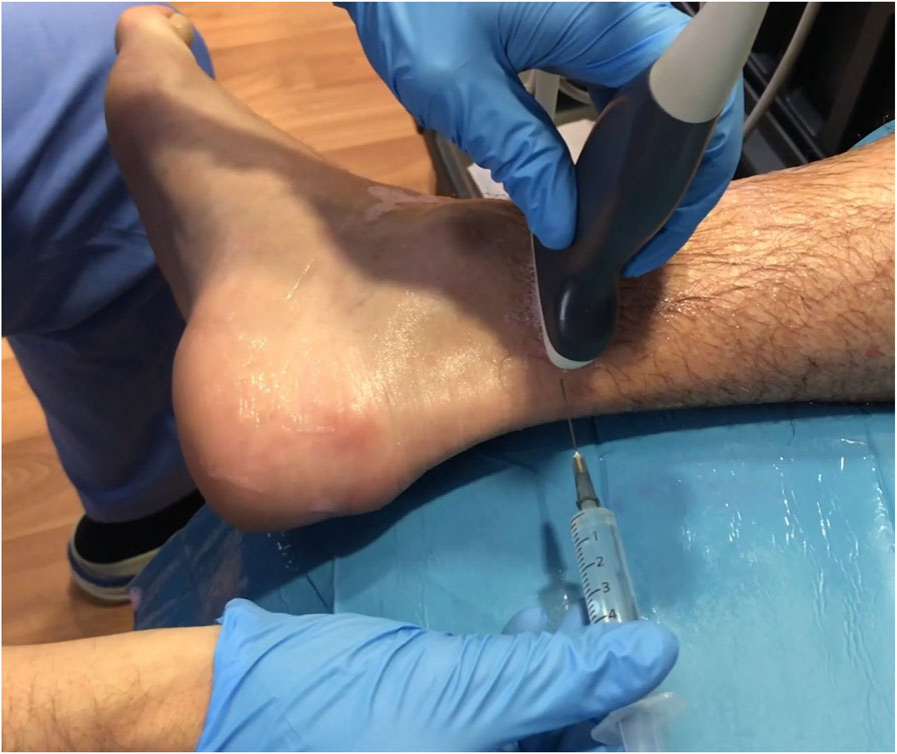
Ultrasound-guided tibial nerve block
